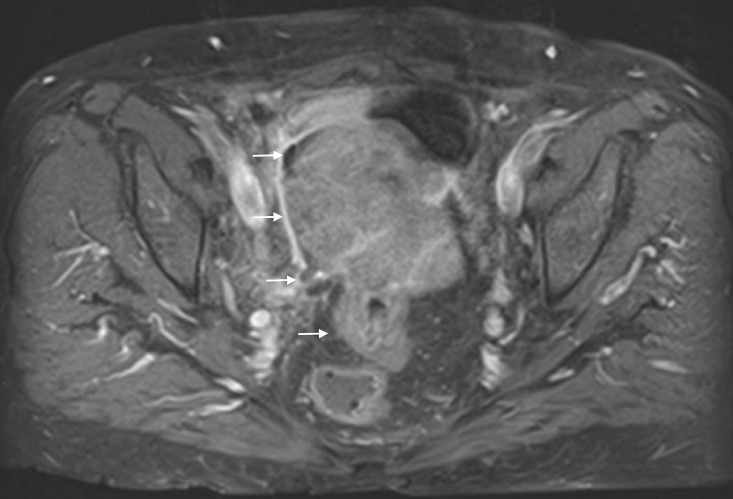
The additional image from the magnetic resonance imaging (MRI) of the abdomen and pelvis, of the ovarian mass, which demonstrates that the mass extends into the colon.
